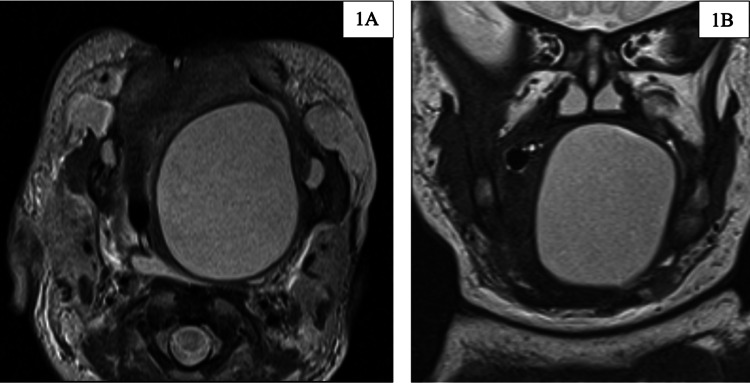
MRI of the oral cavity Axial (1A) and coronal (1B) T2 images of a large, well-circumscribed, non-enhancing cystic lesion in the left tongue base, measuring up to 3.6 cm MRI: magnetic resonance imaging
